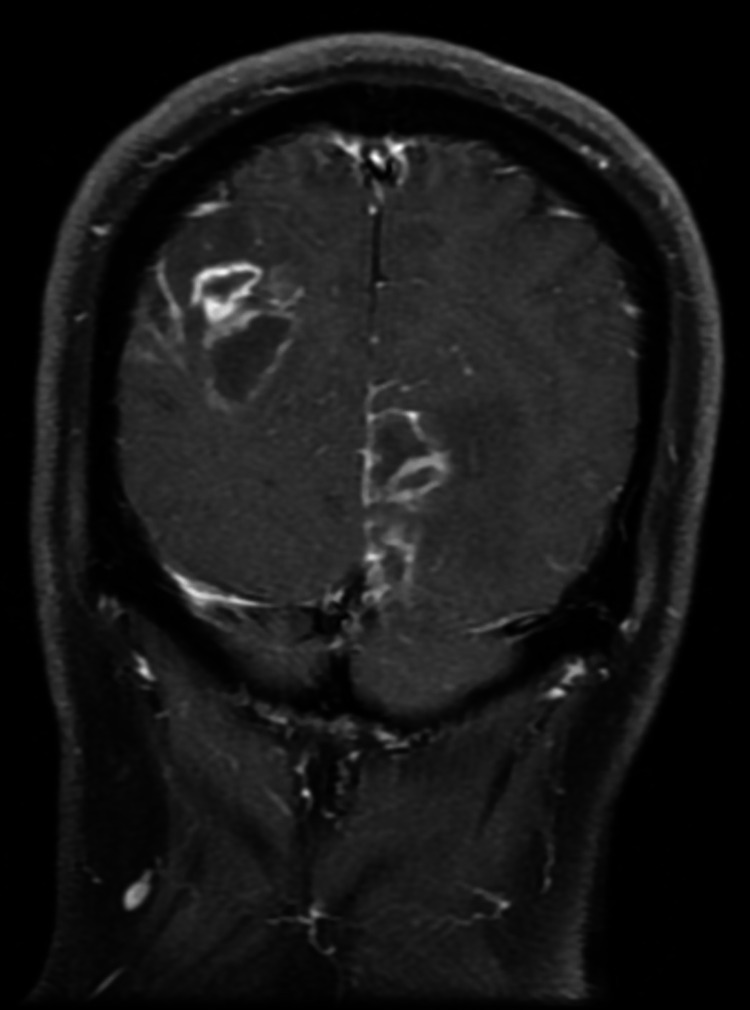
Multiple ring-enhancing lesions noted in a coronal section of brain MRI
